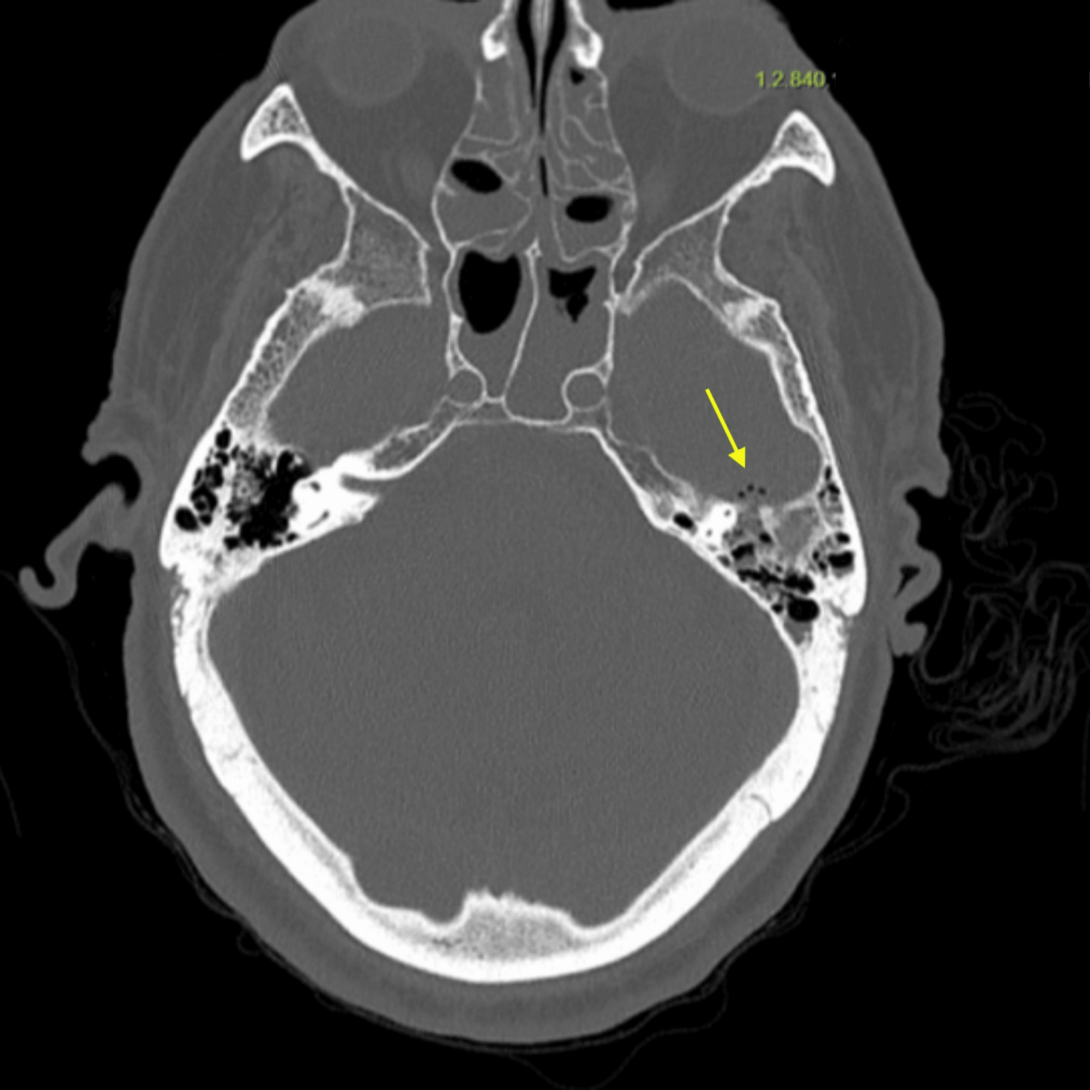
Cranial computed tomography (bone window) Bone-window images showing opacification of the left mastoid air cells and middle ear cavity, with cortical disruption of the anterior wall of the left petrous bone (yellow arrow).
